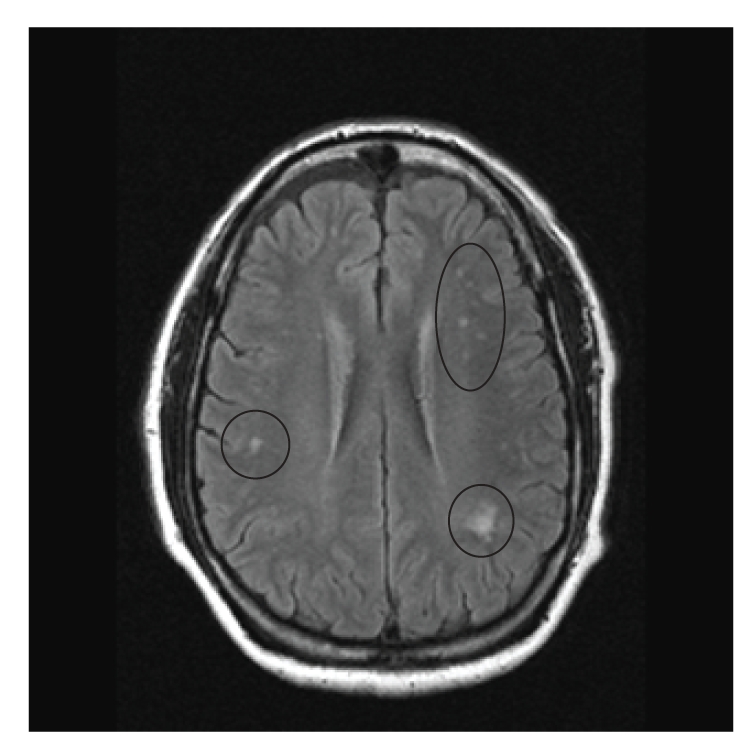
MRI image of the brain in an axial view showing the “precontrast FLAIR image”. Note the abnormal lesions (circled) in the per ventricular area suggesting white matter pathology.
